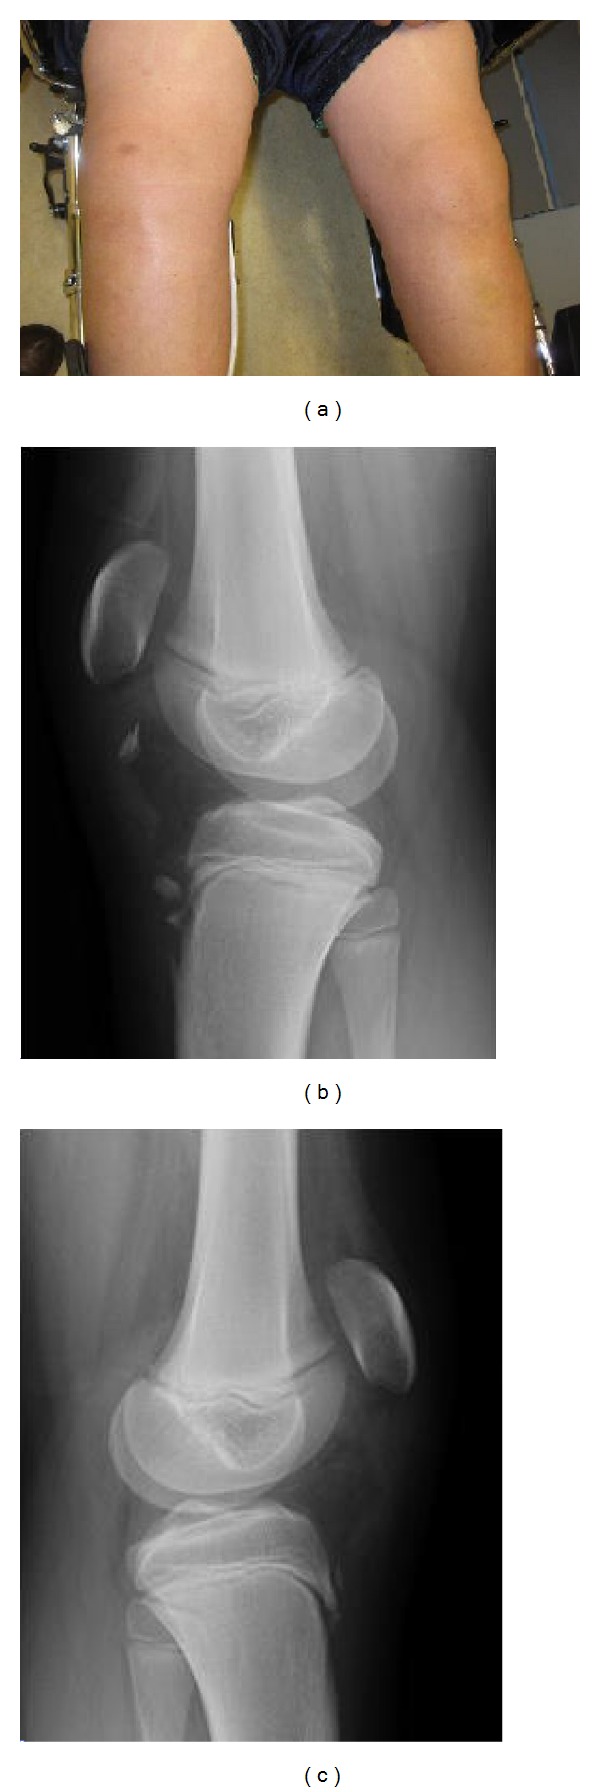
Clinical photograph shows bilateral anterior knee swelling. Bilateral lateral knee radiographs demonstrate the flecks of bone and high-riding patella. Patellar tendon soft tissue shadow shows the waviness of the tendon indicating loss of its integrity.
